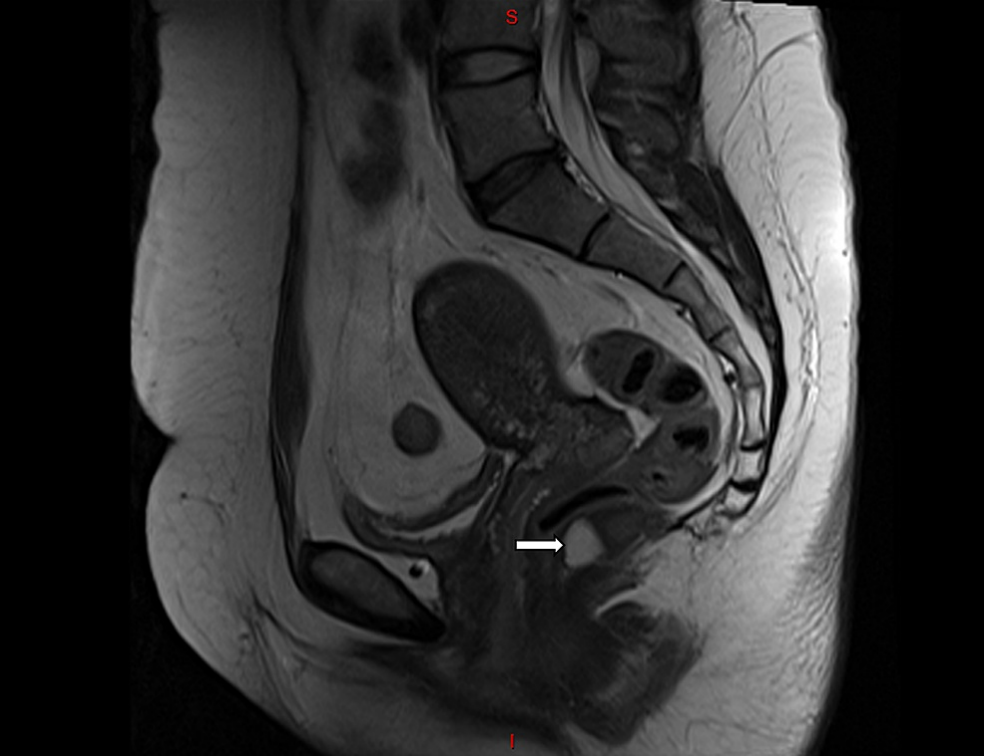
Sagittal MRI of the pelvis: cystic, multi-loculated retrorectal mass (arrow) MRI: magnetic resonance imaging
